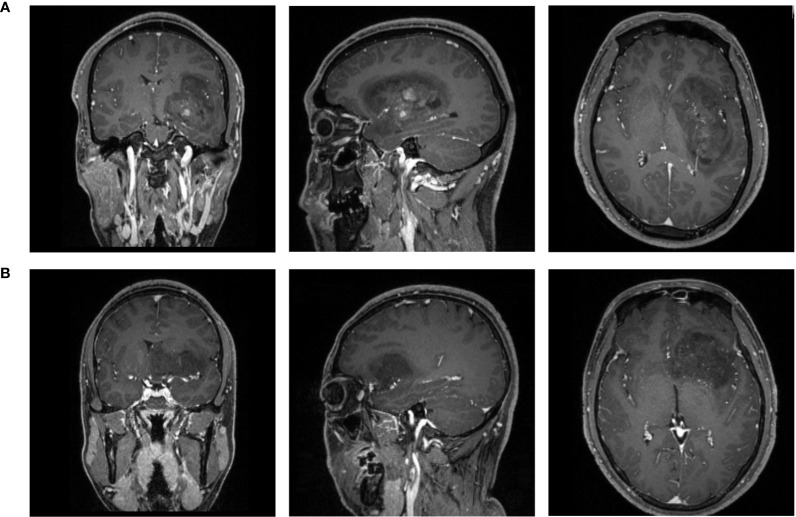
The examples of contrast-enhanced T1 magnetic resonance images of patients with (A) LGA; (B) AA. LGA, low grade astrocytoma; AA, anaplastic astrocytoma.
